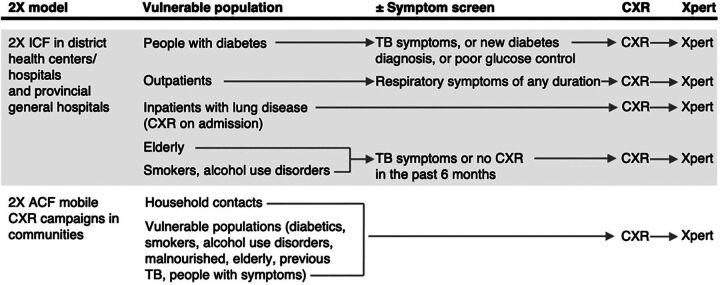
The 2X Health System Strategy to Screen and Diagnose TB Disease,^a^ Vietnam Abbreviations: 2X, Double X; ACF, active case-finding; CXR, chest radiography; ICF, intensified case-finding; Xpert, GeneXpert; ±, with or without. ^a^ Facility-based ICF and community ACF reached a range of vulnerable populations, who were evaluated (with or without symptoms) followed by CXRs, and, if TB-presumptive, Xpert testing.
